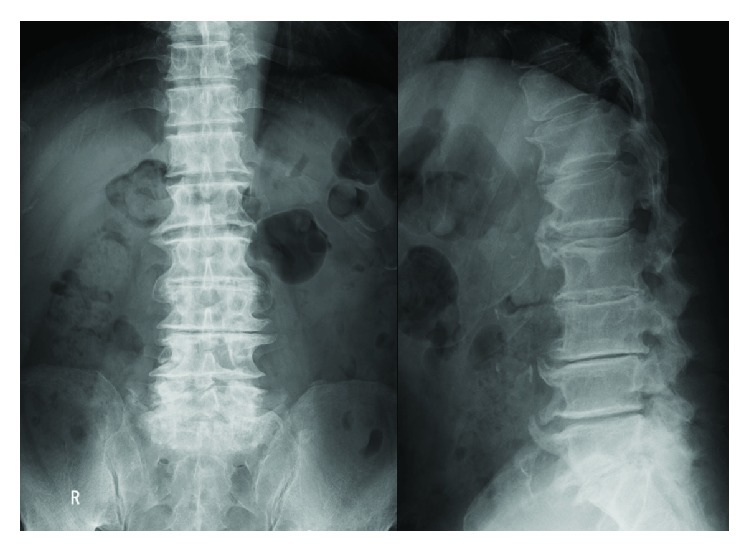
X-ray films of the lumbar spine. Severe spinal degeneration, but no signs of lumbar fracture, was observed.
